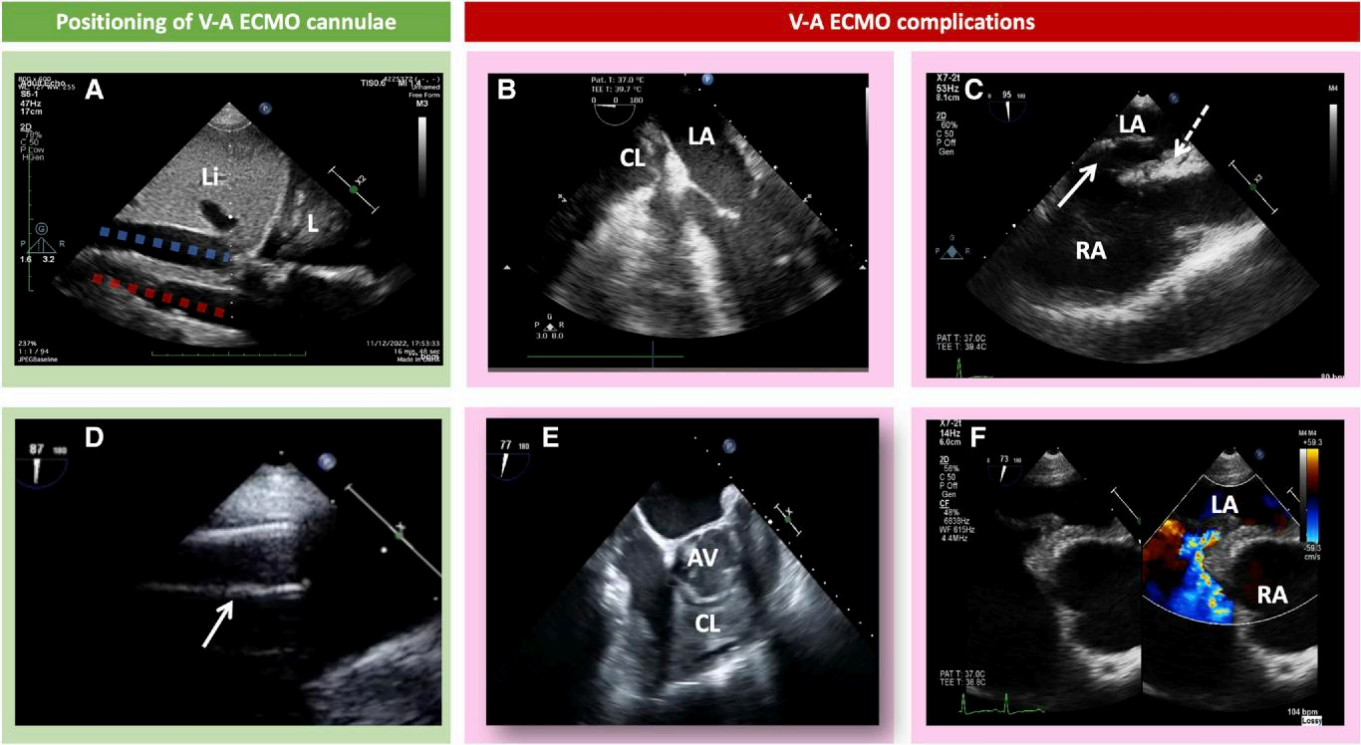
Echocardiography for positioning and troubleshooting during V-A ECMO complications. (*A*) Trans-hepatic mid-axillary window showing the IVC (blue dashed line) and the abdominal aorta (red dashed line) underneath the liver (Li) and the right lung (L). This view is useful for confirming guidewire positioning for both femoral venous and arterial cannula during peripheral V-A ECMO cannulation. (*B*) Mid-oesophageal four-chamber view at 0° showing a collection (CL) compressing the RA, which impaired V-A ECMO flow. (*C*) Mid-oesophageal bicaval view at 95° showing ECMO venous drainage cannula (white arrow) inadvertently invaded the interatrial septum (white dashed arrow). (*D*) Mid-oesophageal bicaval view at 87° showing the ECMO venous cannula (white arrow) tip correctly positioned in the subhepatic IVC. (*E*) Mid-oesophageal RV inflow–outflow view at 77° showing a clot (CL) in the RA extending into the PA in a patient on peripheral V-A ECMO. (*F*) Colour Doppler of the same window and same patient in *C* showing interatrial shunt induced by inadvertent venous cannula malpositioning, which infiltrated the interatrial septum and led to an iatrogenic interatrial septal defect. The patient required IAS defect closure with a septal closure device before V-A ECMO weaning.
